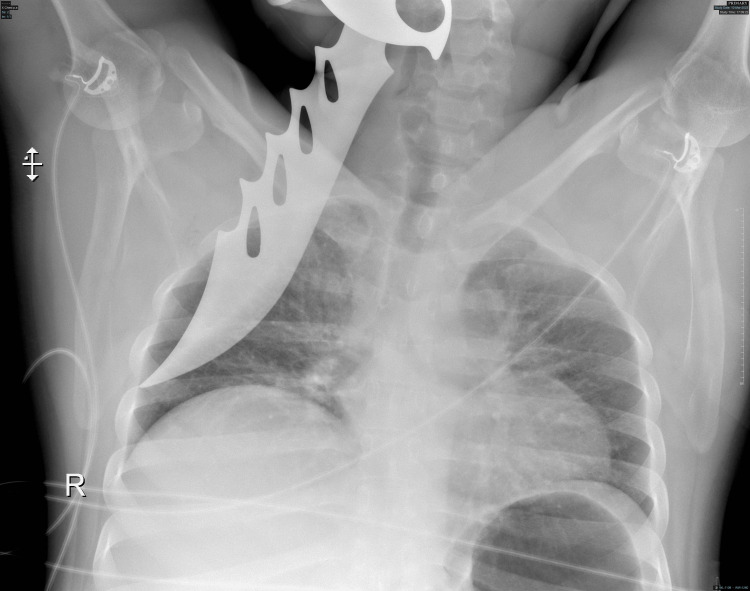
A chest X-ray showing an impaled knife on the right side of the chest.
